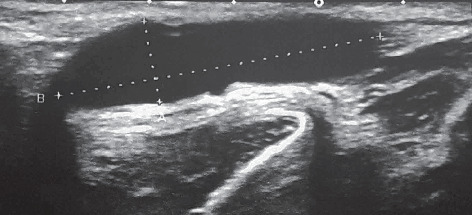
Autovenous graft dilated (an ultrasound image).
